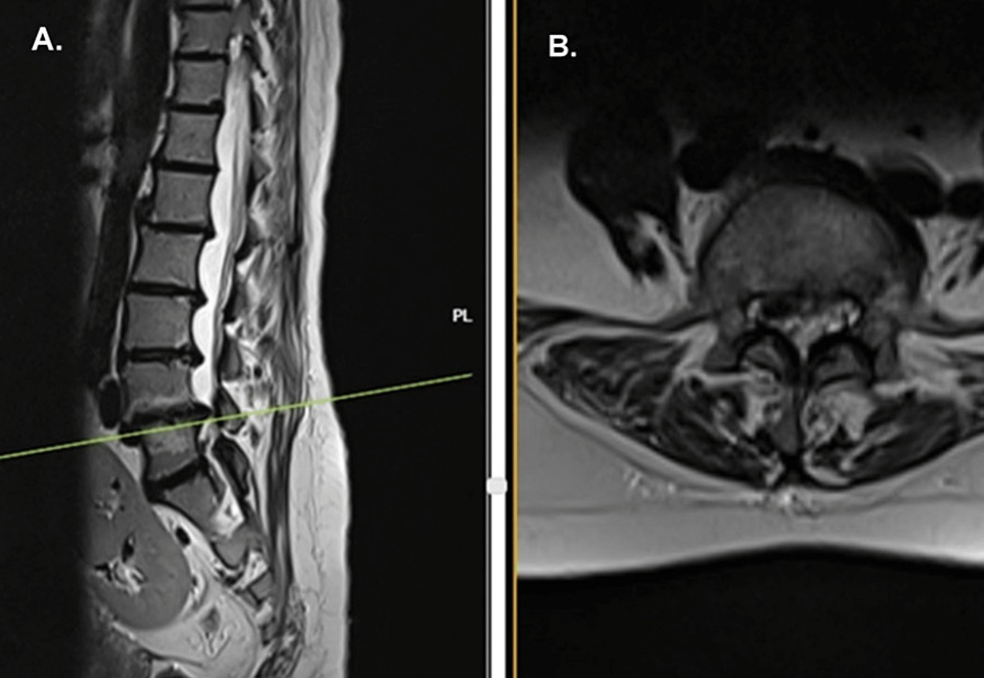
MRI sagittal view (A) and axial view (B) showing a prolapsed disc at the L4-L5 level extending to the right side and causing symptoms
